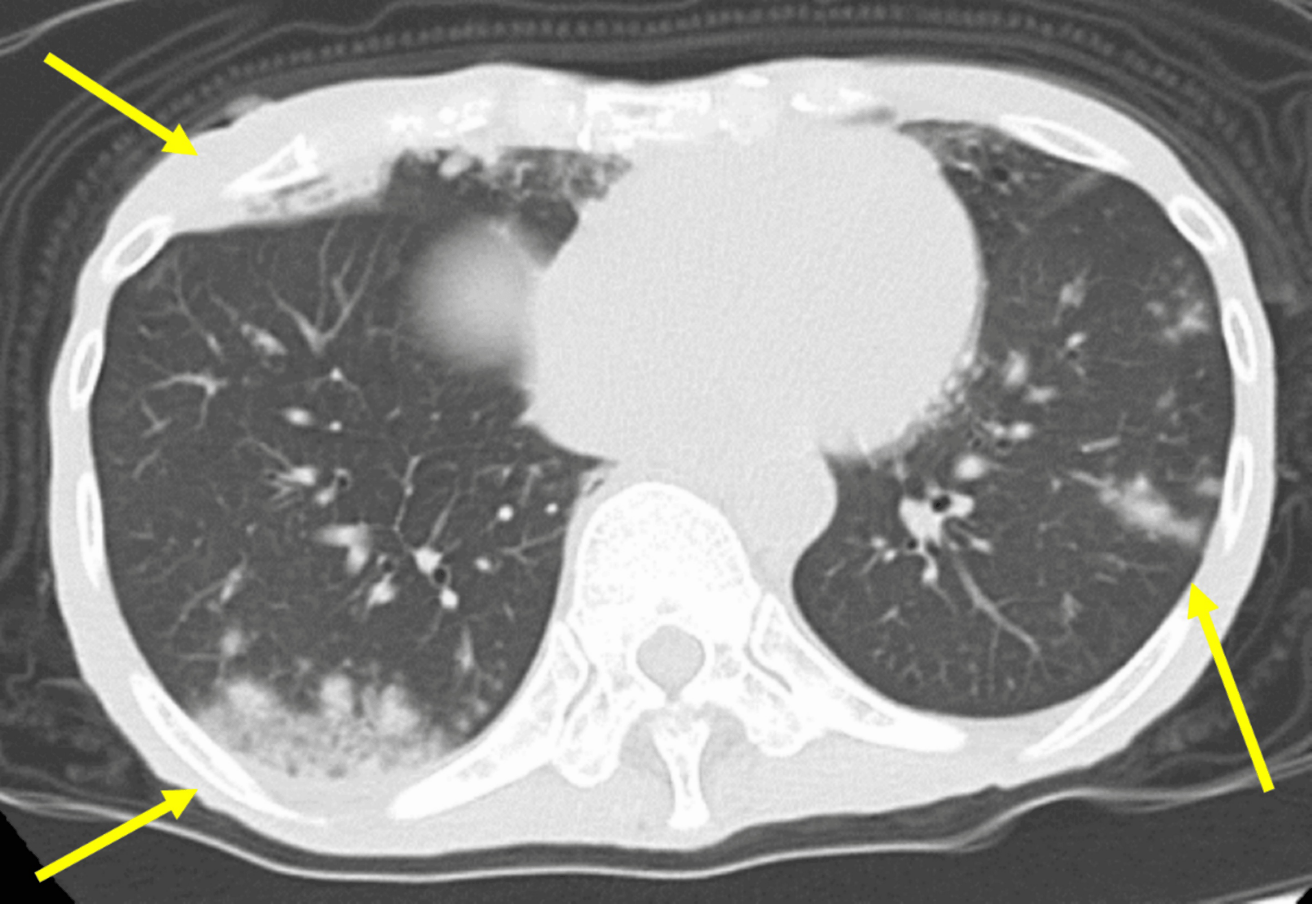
Chest CT on admission shows pneumonia.
